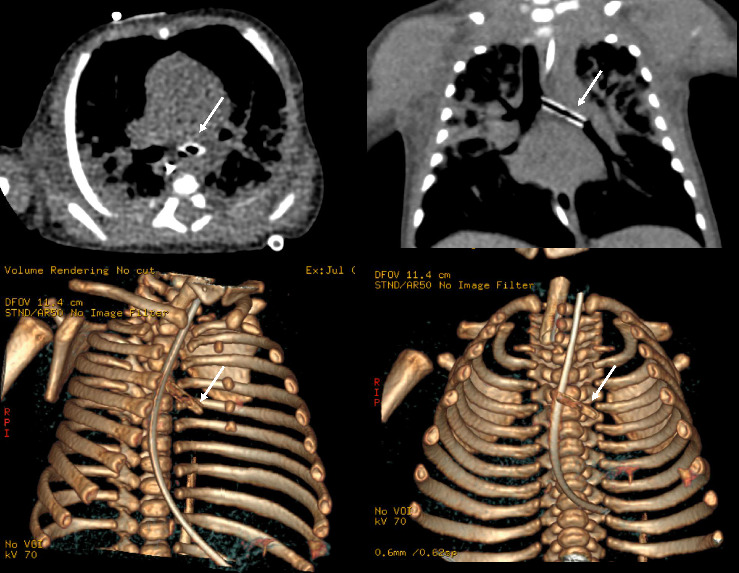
CT scan with 3D remodeling. White arrow indicates cylindrical foreign body.
